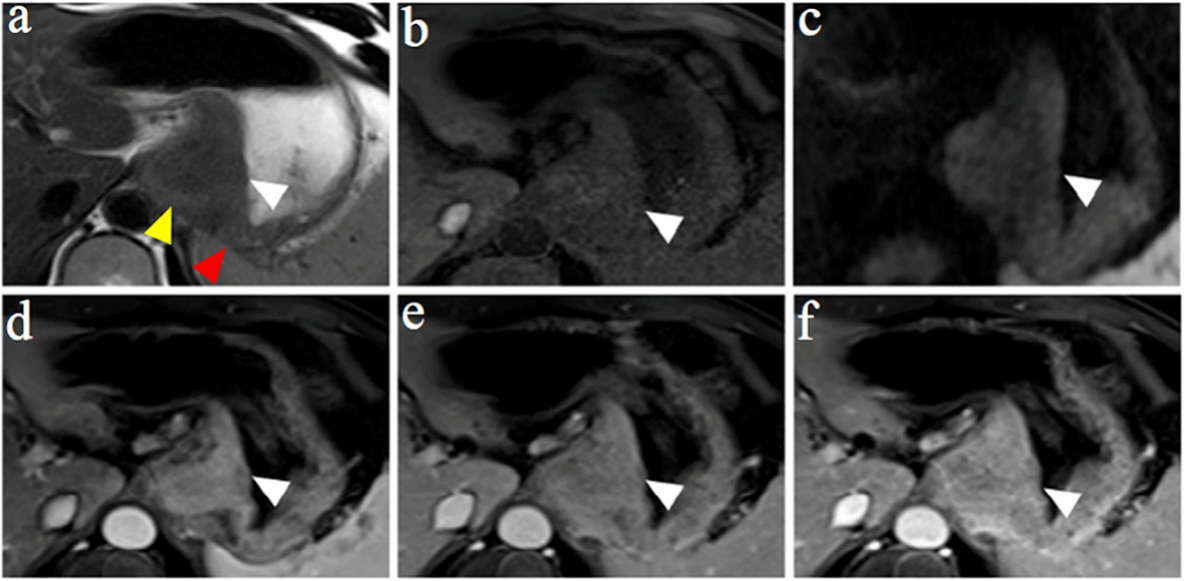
A 48-year-old man with esophagogastric junction cancer was pathologically diagnosed using HR MRI as having stage T4b, Sierwert II. a The axial T2W image shows clear thickening of the cardia wall, and the invasion of the left diaphragmatic crus and spleen was also observed (white, yellow and red arrows, respectively). b The axial diffusion-weighted image with b = 800 s/mm^2^ showing hyperintensity in the cardia wall. c An inhomogeneous thickening pattern of the cardia wall is shown on the axial T1W image. d–f The lesion was markedly enhanced and invaded into the left diaphragmatic crus and spleen on arterial, venous and delayed phases of the enhanced T1W images
